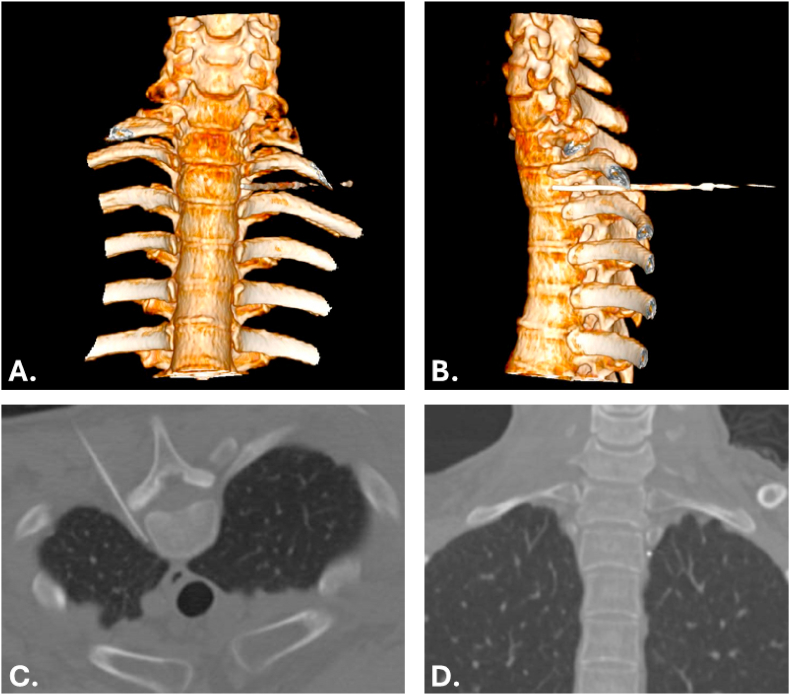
CT-guided TSB. A, Three-dimensional volume-rendered anterior view. B, Three-dimensional oblique/lateral view showing the needle trajectory. C, Axial CT image at the intervention level. D, Coronal visualization of the needle tip position.
